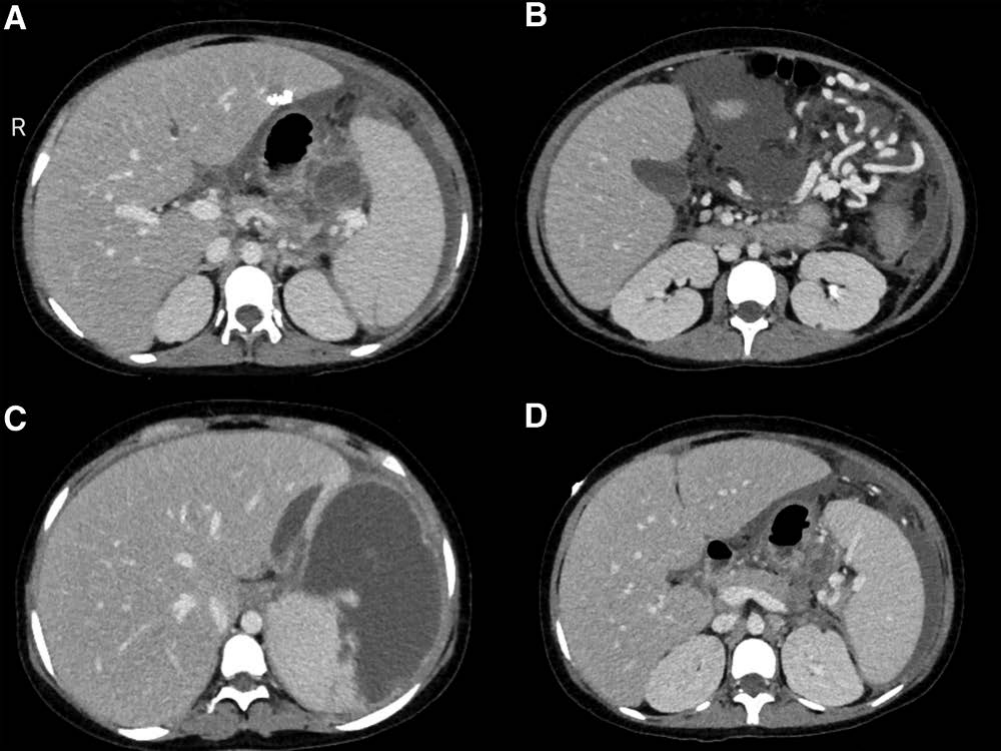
Abdominal CT of the proband: (a) Enlargement of liver and spleen. (b) Multiple varices in the left upper abdomen. (c) Cystic mass in the anterior part of the spleen.(d) The changes of chronic pancreatitis, suspected splenic vein obstruction. CT = computed tomography.
